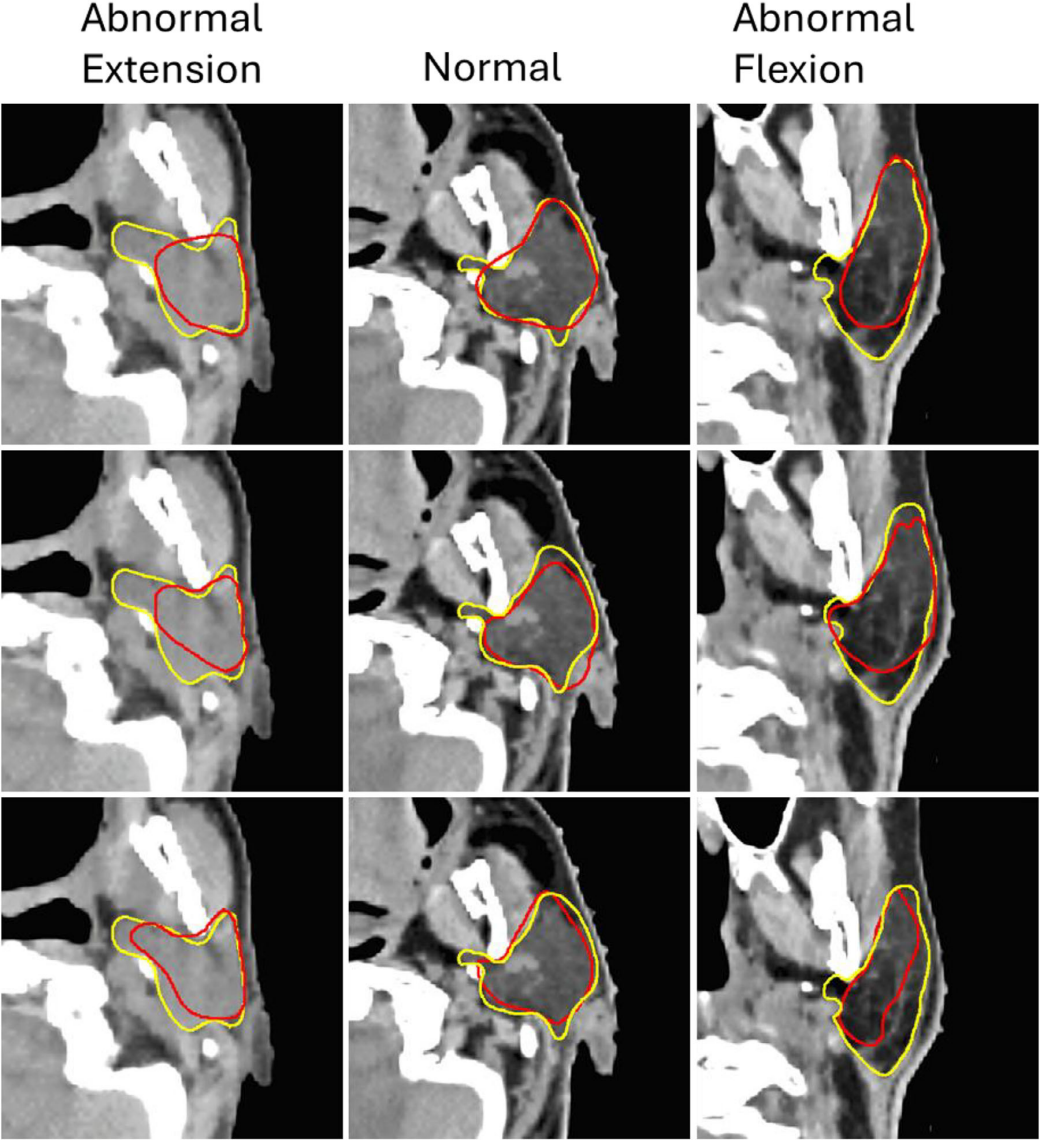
CT images with gold standard parotid reference contours (yellow) and vendor reference contours (red) for vendors 5, 7, and 3 (top to bottom) are shown. Neck angles are −16°, 11.5°, and 66° (left to right).
